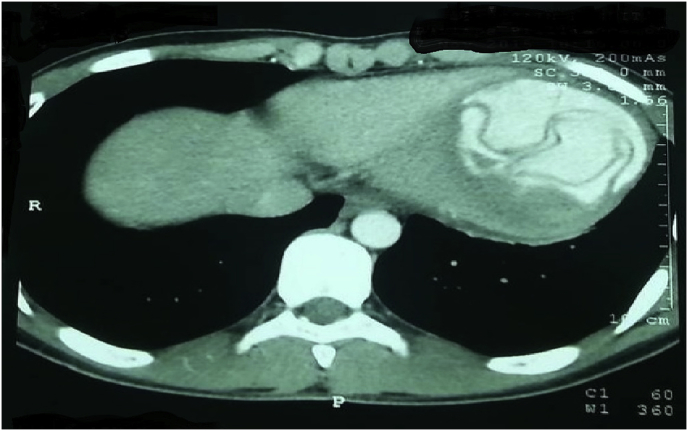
CT Scan show laminated membrane ruptured LV Hydatid cyst.
